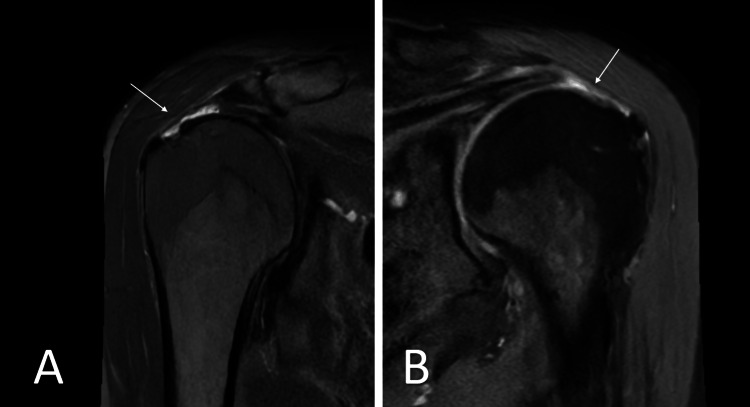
Magnetic resonance imaging (MRI) of both shoulders A) MRI T2 weighted images with fat suppression of the right shoulder in the coronal plane revealing the rupture of the supraspinatus tendon (arrow) with a retraction of approximately 2cm. B) MRI protein density weighted images in the coronal plane of the left shoulder showing the supraspinatus tendon tear with a retraction of approximately 1.8 cm.
